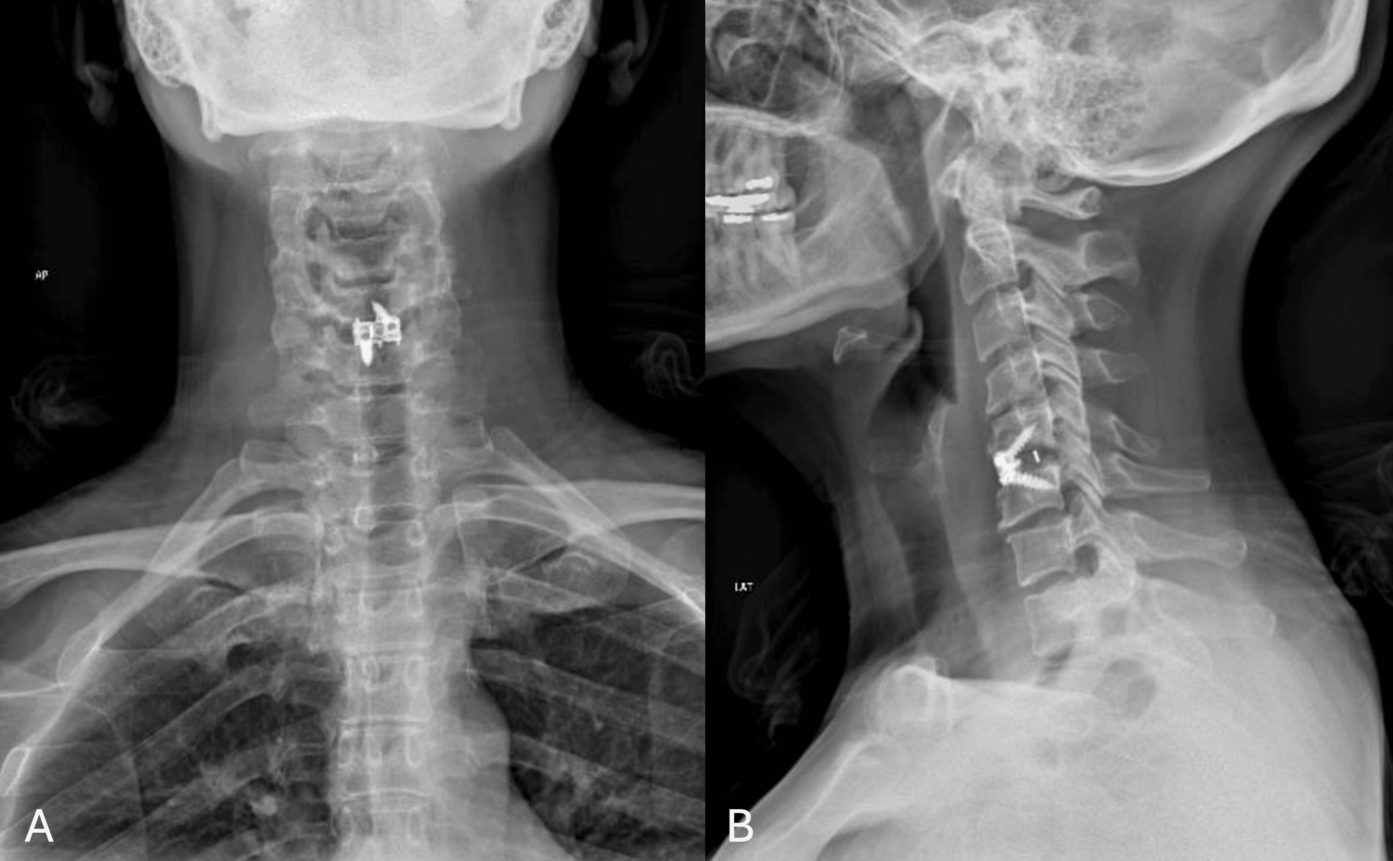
Postoperative cervical radiographs. (A) Anteroposterior view showing appropriate placement of a C5-C6 stand-alone cage. (B) Lateral view demonstrating correct cage positioning with maintained overall cervical alignment, although mild straightening of the cervical lordosis is noted.
